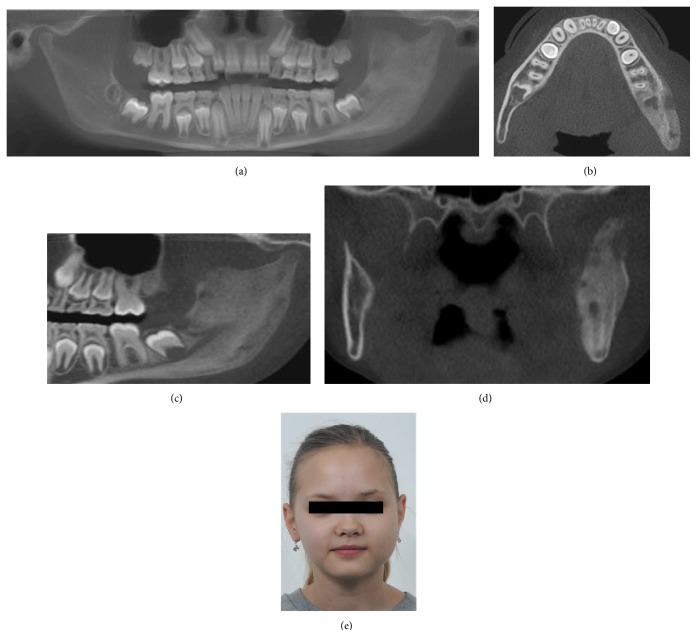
Preoperative CBCT and clinical image of patient case 1 with PCO. (a) Panoramic CBCT; (b, c, and d) axial, sagittal, and coronal sections of sclerotic mandible with new bone formation and lytic lesion at left posterior site; and (e) patient photo with swelling of the left side of the face.
